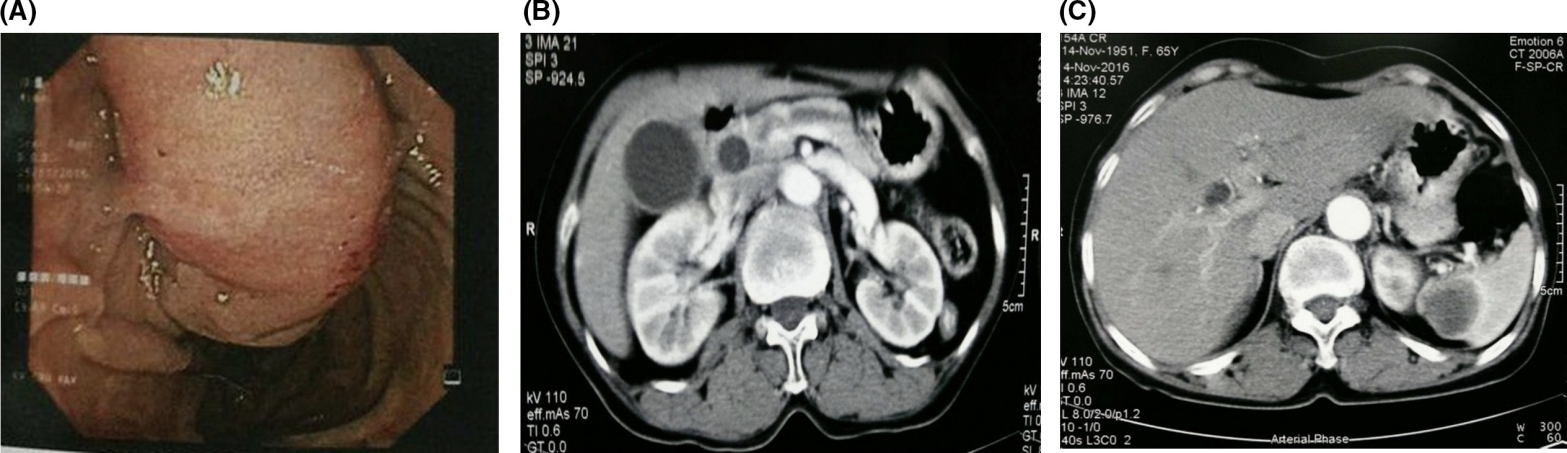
Image diagnostics before surgery. (A) The duodenoscopy revealed an ampullary tumor. (B) The abdominal computed tomography scan images showed dilation of both the internal and external hepatobiliary tract; Wirsung's diameter was 5 mm. (C) The hypodense lesion in the spleen was 3 cm.
